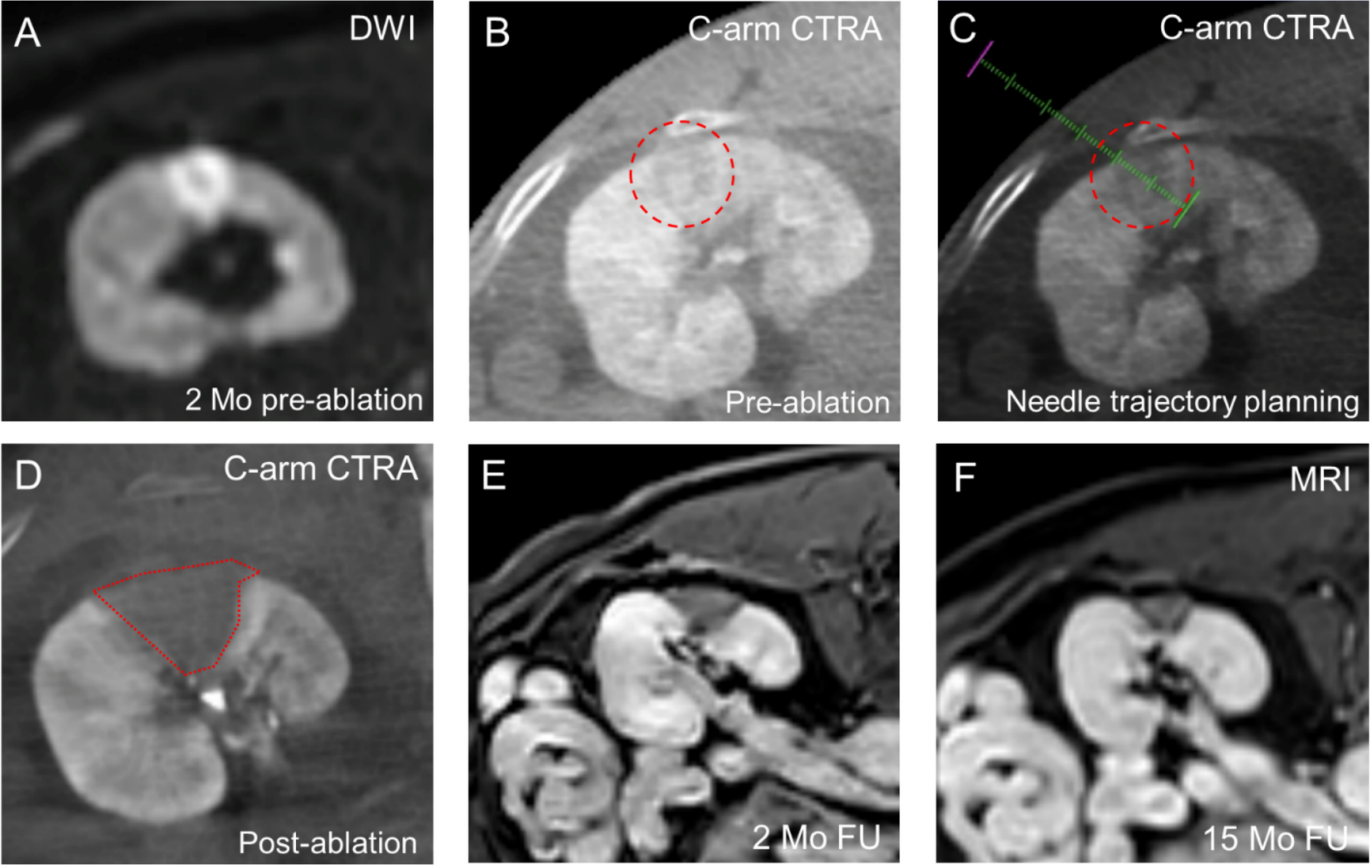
Example of a RenACAGA procedure of an intraparenchymal tumor (15 mm) in the left kidney. A The lesion is visualized on diffusion-weighted imaging (DWI) 2 months prior to the ablation procedure; B Intraprocedural C-arm CT renal arteriography (C-arm CTRA) depicts the target lesion (red circle); C Needle trajectory planning is performed using XperGuide software (Philips) to accurately target the tumor (red circle); D The ablation zone (outlined in red) after 2 min ablation at 100W is depicted with C-arm CTRA immediately after ablation; E, F Follow-up (FU) MRI (T1 with gadolinium) at 2 months and 15 months demonstrates no local tumor recurrence and a progressively shrinking ablation zone
